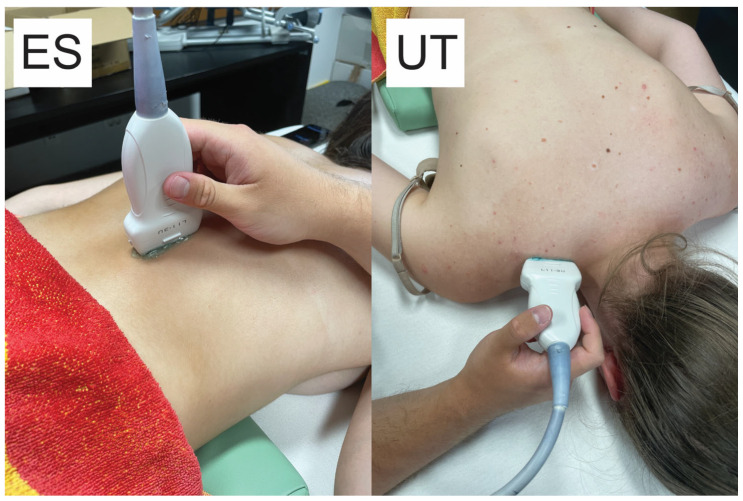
Snapshots of the ultrasound measurements showing the probe positioning on a participant. ES, erector spinae; UT, upper trapezius.
